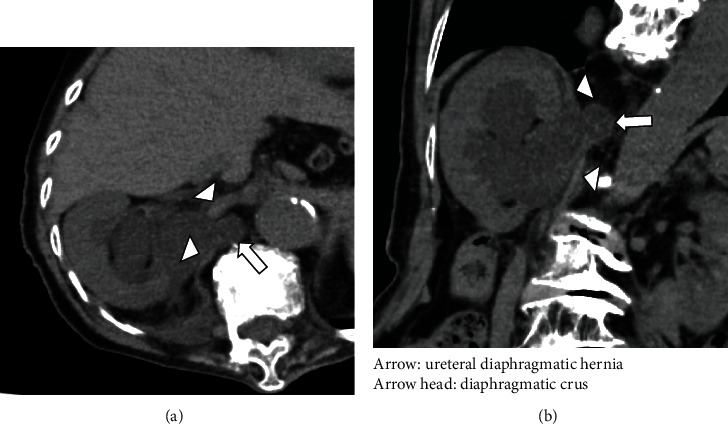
CT of the abdomen shows a diaphragmatic hernia of a part of urinary tract resulting in moderate-severe hydronephrosis ((a) axial and (b) coronal).
